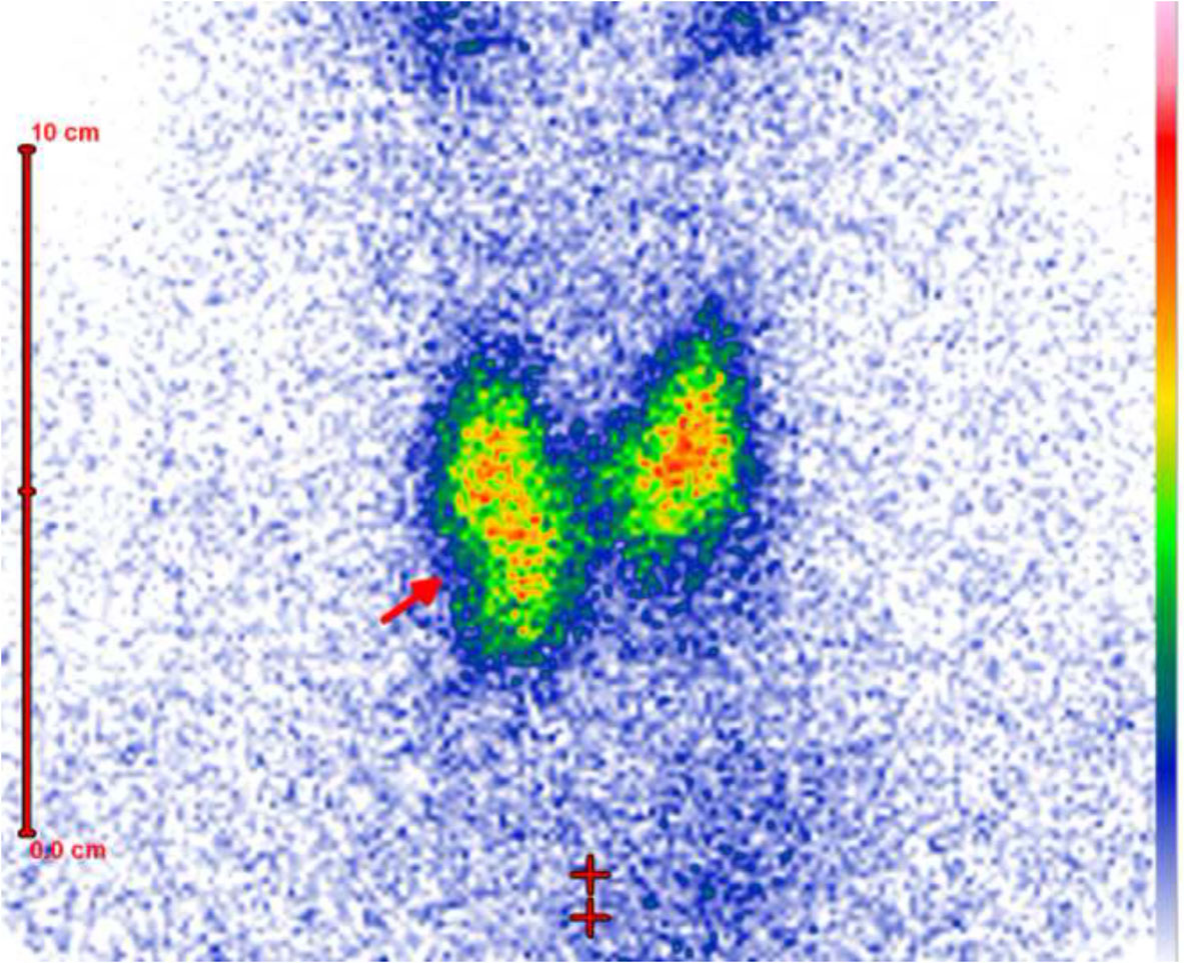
TS shows an area with diminished uptake in the right lobe of the thyroid laterocaudal which is consistent with a cold nodule. Abbreviations: TS, Thyroid scintigraphy
